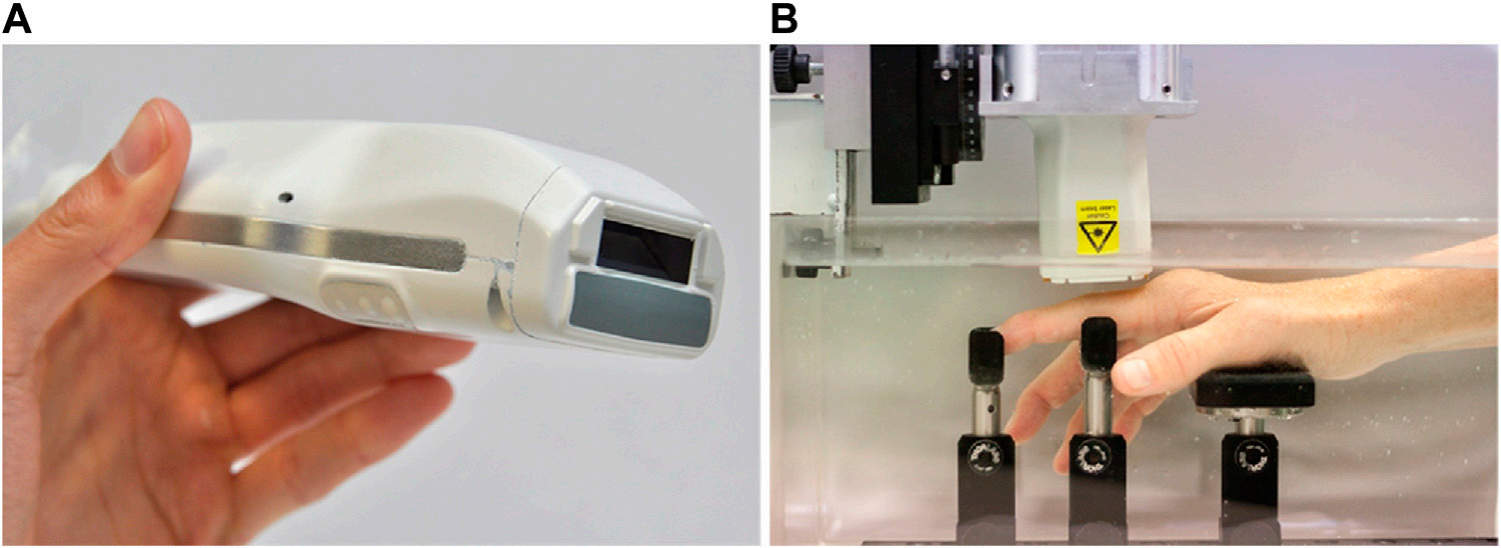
(A) A PAT/US probe with a front-end view showing the light delivery window (dark aperture) and gray acoustic lens in medium; (B) The patient’s hand is submerged in water and is supported by a series of braces. The sensor is mounted on a motorized 2-axis stage and positioned above the joint. PAT: photoacoustic tomography; US: ultrasound. Reprinted with permission from [van den Berg P. J, Daoudi K., Bernelot Moens H. J., and Steenbergen W. (2017). Feasibility of photoacoustic/ultrasound imaging of synovitis in finger joints using a point-of-care system. Photoacoustics. 8,8–14. doi: 10.1016/j.pacs.2017.08.002].
